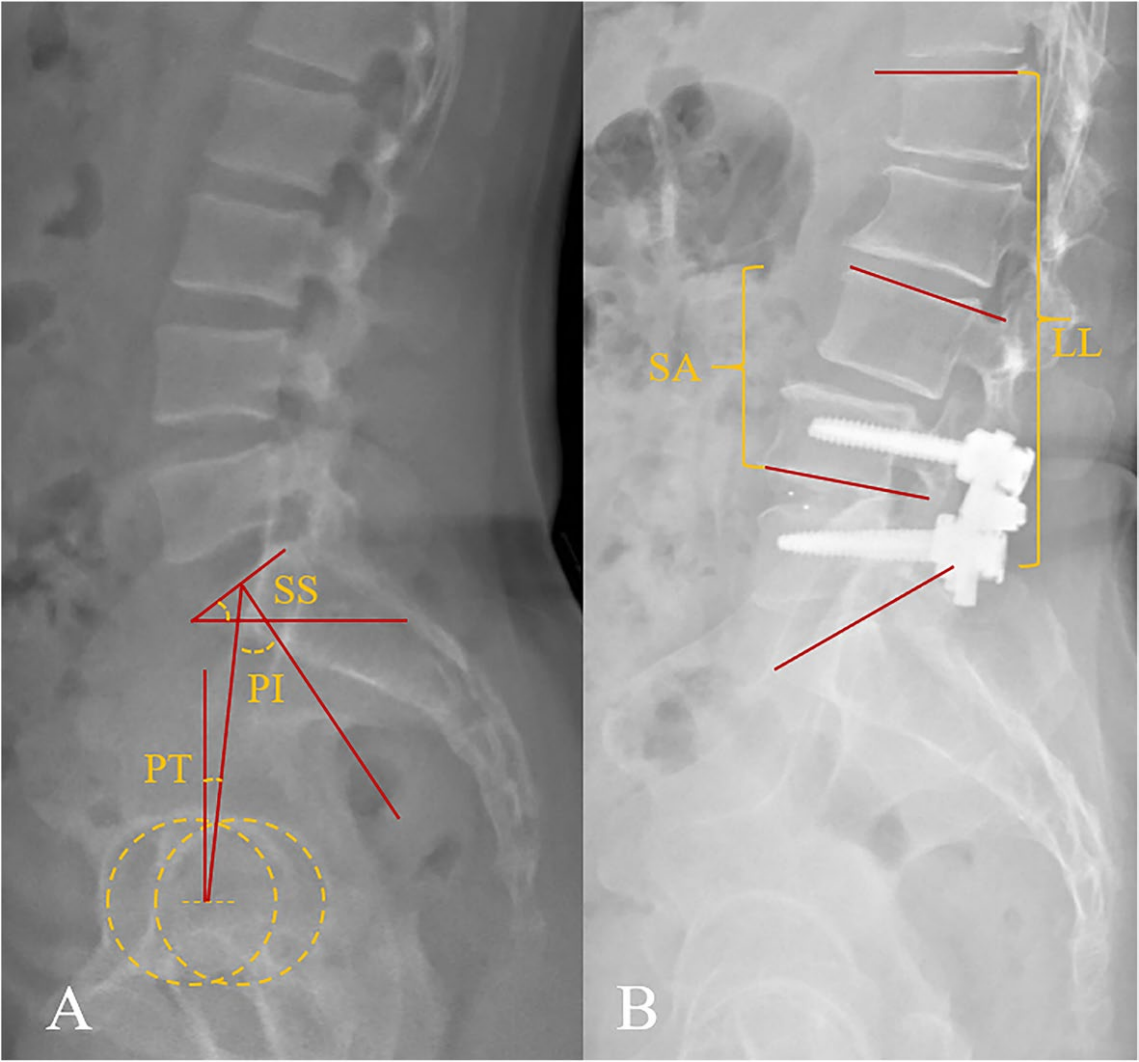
Measurement of radiographic parameters. A. spinopelvic parameters: Pelvic Incidence (PI) is measured as the angle between the line perpendicular to the middle of sacral plate and the line connecting the hip axis and midpoint of sacral plate; Pelvic Tilt (PT) is the angle between the vertical line and the line joining hip axis and the middle of sacral plate; Sacral Slope (SS) is formed by the endplate of S1 and the horizontal line. B. Lumbar Lordosis (LL) is regarded as the angle between the upper endplates of L1 and S1, using the Cobb method; Segmental Angle (SA) is defined by the Cobb angle between the upper endplate of the cephalad adjacent vertebra and the lower endplate of the upper instrumented vertebra
